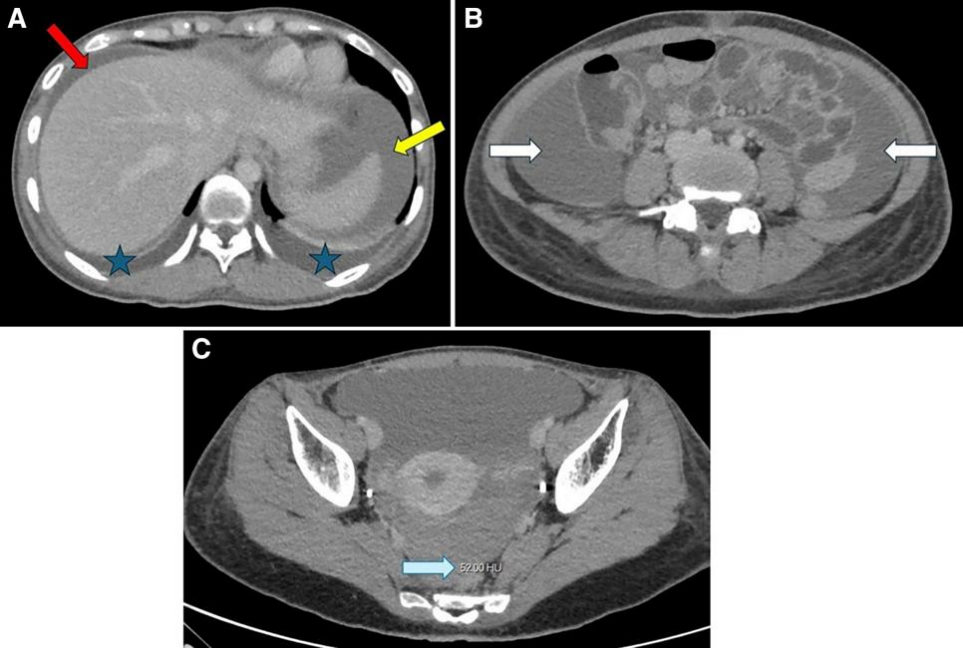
Contrast-enhanced computed tomography images show a large volume of hemoperitoneum in (A) the perihepatic region (red arrow), (A) the perisplenic region (orange arrow), and (B) the bilateral paracolic gutters (white arrows). (A) Bilateral pleural effusion is noted in both lungs (blue star). The liver and spleen appear normal. (C) The pelvic cavity has a region of interest measurement of 52 Hounsfield units (HU; blue arrow).
